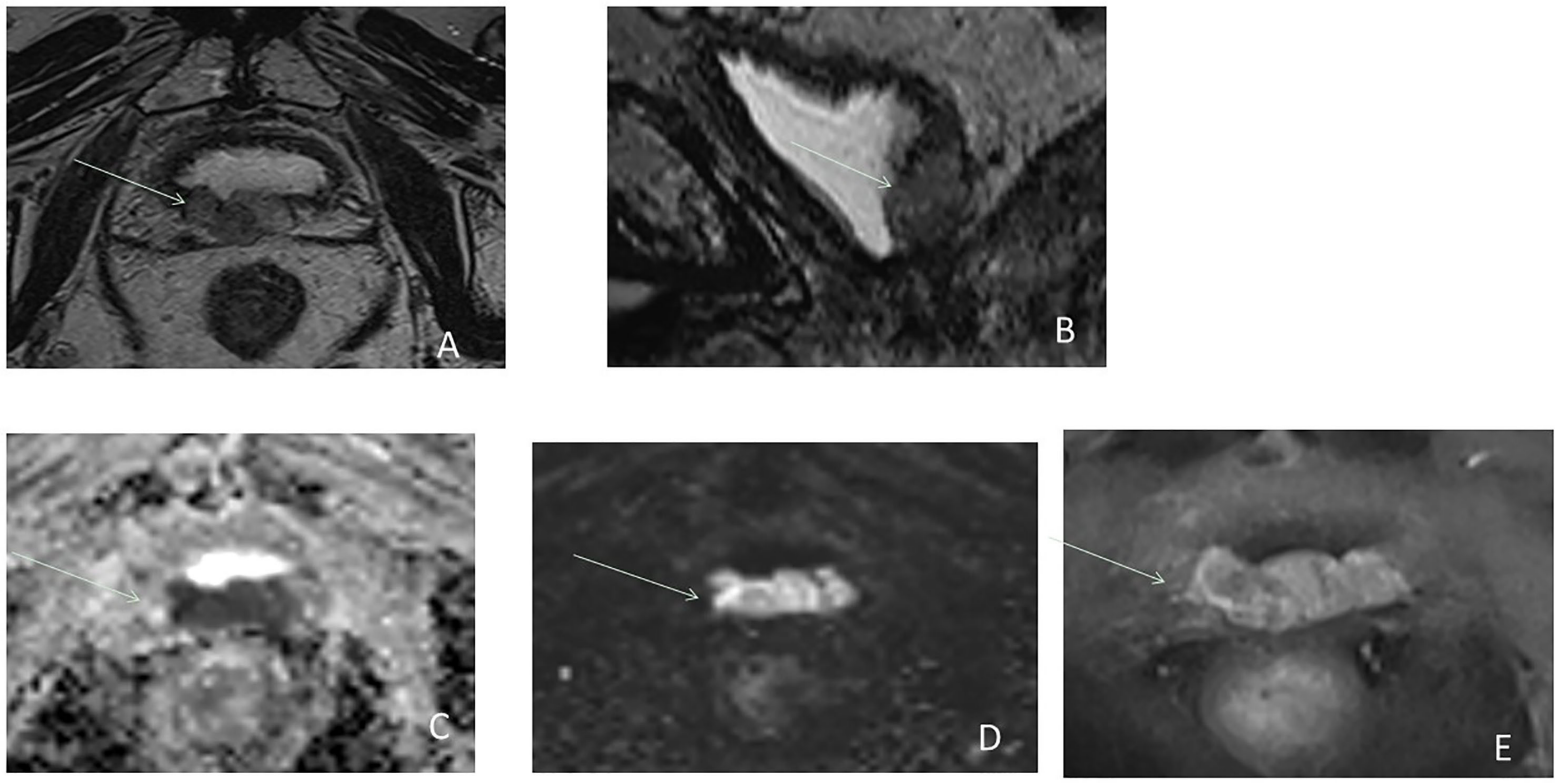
Postsurgical lesion recurrence (arrow) in a 63-year-old male with serum PSA = 0.8 ng/ml. Axial T2W MRI (A), sagittal T2W (B), ADC map (C), b2000 DW MRI (D), and DCE MRI (E) show a large lobulated mass relatively hyperintense on T2W, hypointense area on the ADC map, hyperintense area on b2000, and with early hyperenhancement located in the postpart of the bladder neck.
